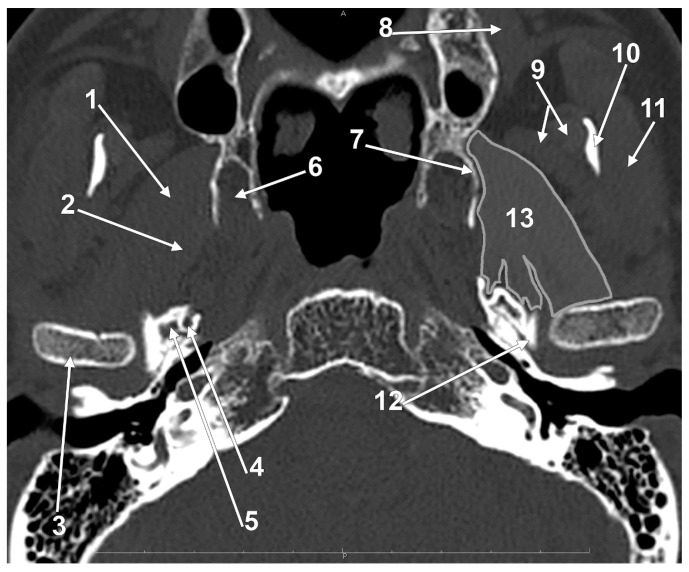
Axial CT slice through the upper parts of the pterygoid plates. Inferior view. Pterygospinous bundles of the lateral pterygoid muscles: 1. inferior head of the right lateral pterygoid muscle; 2. pterygospinous muscle; 3. head of the mandible; 4. spinous foramen; 5. sphenoidal spine; 6. medial pterygoid muscle; 7. lateral pterygoid plate; 8. buccinator muscle; 9. temporal muscle; 10. coronoid process; 11. masseter muscle; 12. endoglenoid process; and 13. inferior head of the left lateral pterygoid muscle.
